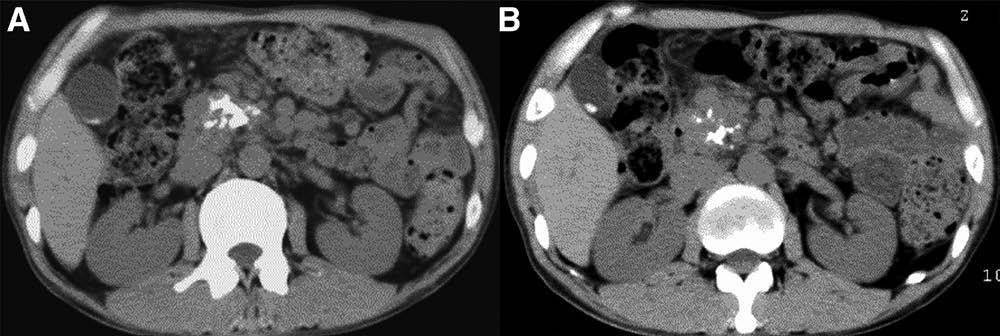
CT findings before and after nonsurgical treatment for pancreatolithiasis without abdominal pain. (A) Before nonsurgical treatment, CT showed stones with molded contours and diameters up to 20 mm in the duct within the pancreatic head. B. After nonsurgical treatment, CT showed disappearance of stones from the pancreatic head. CT = computed tomography.
